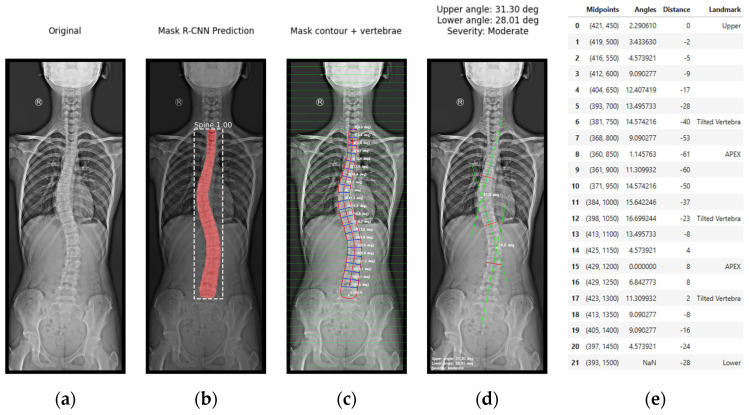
Visualization of the stages in JupyterLab: (a) original anteroposterior (AP) full spine X-ray image; (b) Mask R-CNN spine segmentation; (c) mask contour and vertebral angles; (d) computed Cobb angle, and severity classification (red lines correspond to the most tilted vertebrae, while green lines represent the perpendiculars used in the Cobb angle calculation); (e) data table displaying numerical values and anatomical landmarks.
